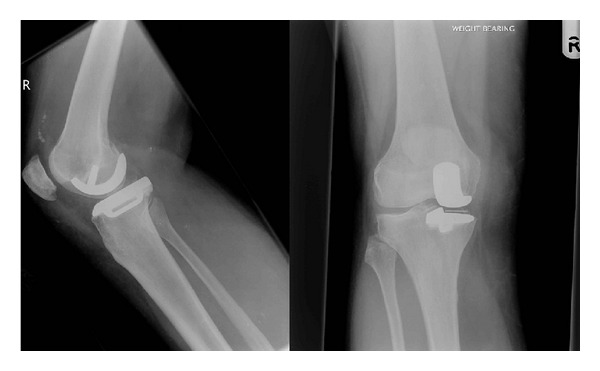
Lateral view and AP view radiograph of the knee taken following operation.
